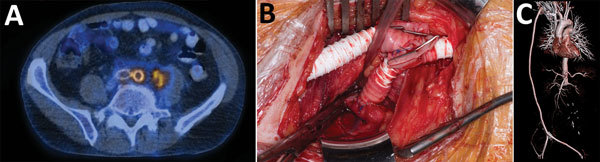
Preoperative, intraoperative, and postoperative images in the case of a patient who received an abdominal aortic endograft and was later diagnosed with *Mycobacterium chimaera* and *Granulicatella adiacens* infection, Switzerland, 2014. A) ^18^Fluorodeoxyglucose positron emission tomography–computed tomography scan at diagnosis indicating a strong, metabolically active (maximum standard uptake value 9.7) aortic endograft infection and an adjacent abscess formation in the iliopsoas in close contact with the left common iliac artery. B) Intraoperative extra-anatomic position of a polytetrafluoroethylene graft through noninfected subcutaneous operative field. C) Satisfactory postoperative result of the axillo-bifemoral bypass on volume-rendered reconstructions of a contrast-enhanced computed tomography.
